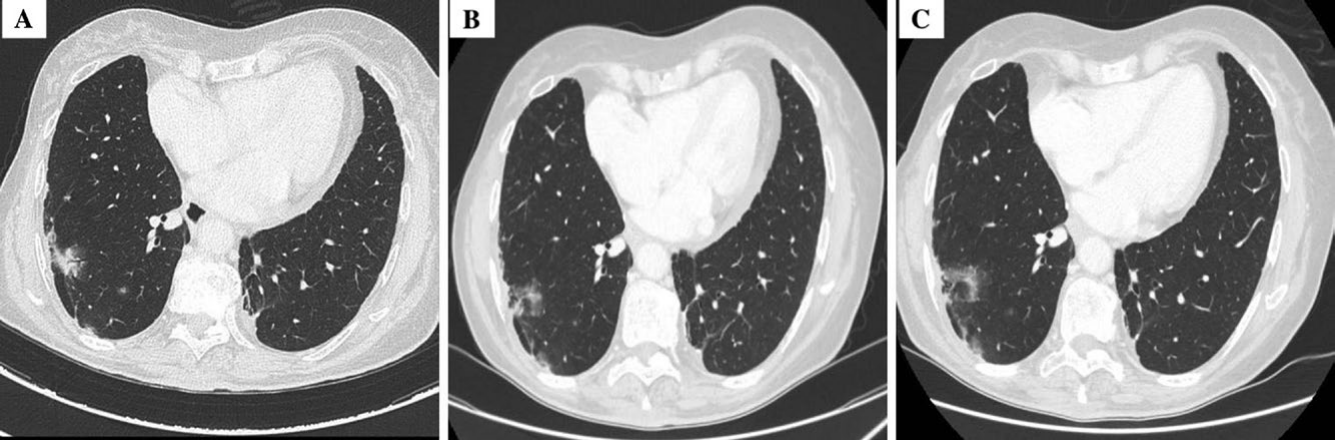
CT-scan sections focusing on the largest measurable lesion in the upper lobe of the right lung. (a) Disease progression on March 2024. (b) Restored tumor response after Sotorasib dose re-escalation in July 2024. (c) Confirmed tumor response on October 2024. Abbreviation: CT, computed tomography.
